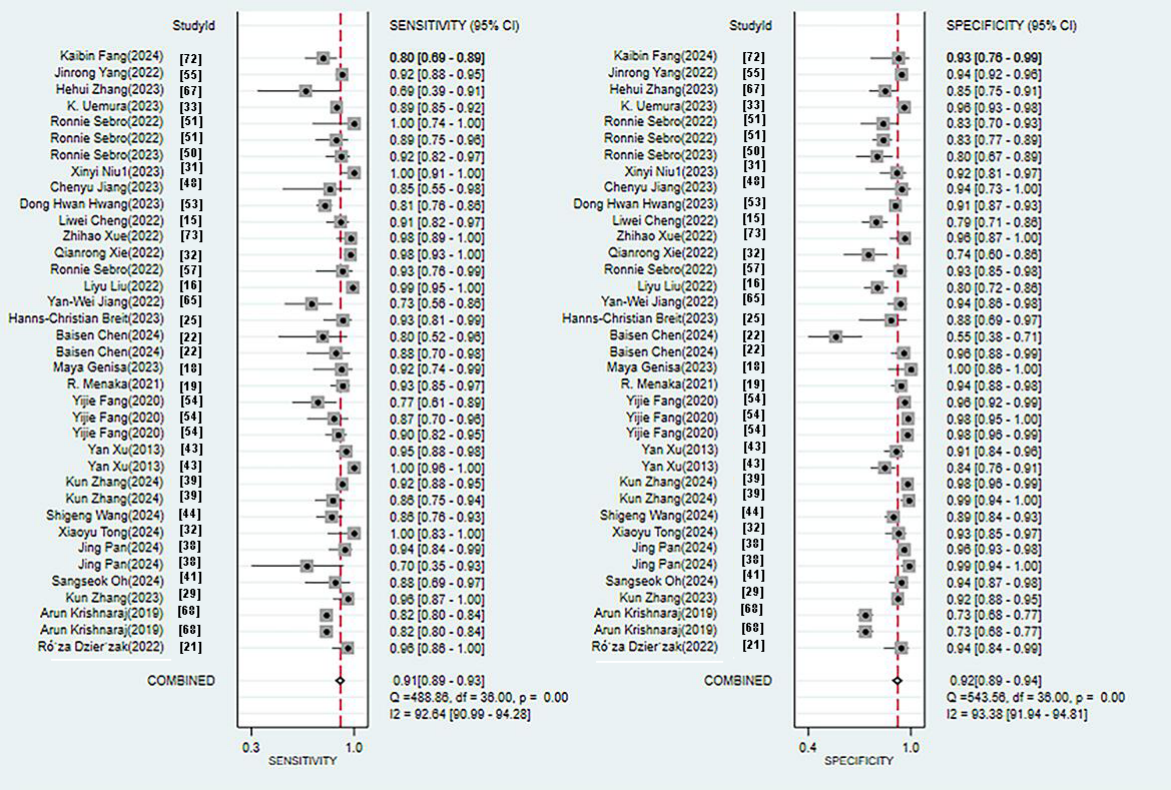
Forestplot of sensitivity and specificity for computed tomography-based machine learning for diagnosing osteoporosis [15,16,18,19,21-23,25,26,29,31-33,38-41,43-45,47,48,50-54,56-58,65,67-69,72,73].
